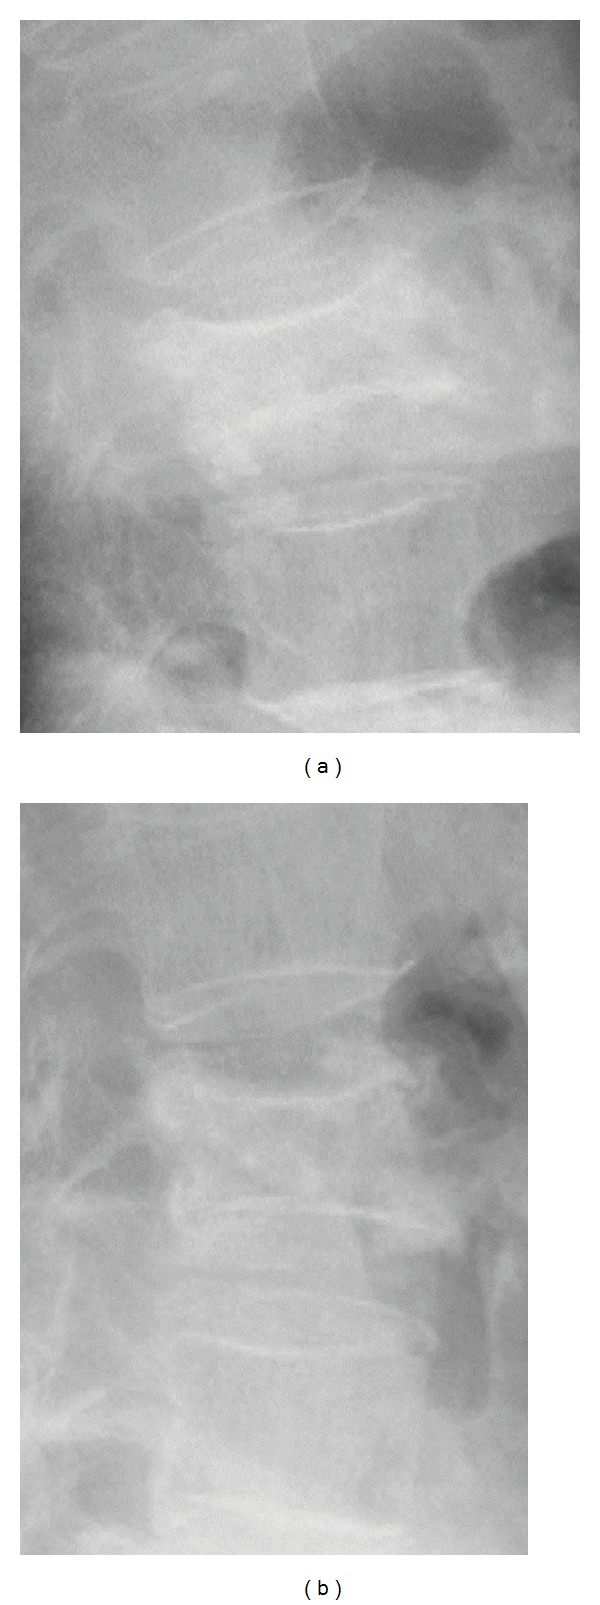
Lateral radiographs (a) taken in standing and (b) supine positions after four months of conservative treatment of an 85-year-old woman with an L3 vertebral body fracture show the L3 vertebral body more collapsed in the standing (a) than in the supine position (b).
